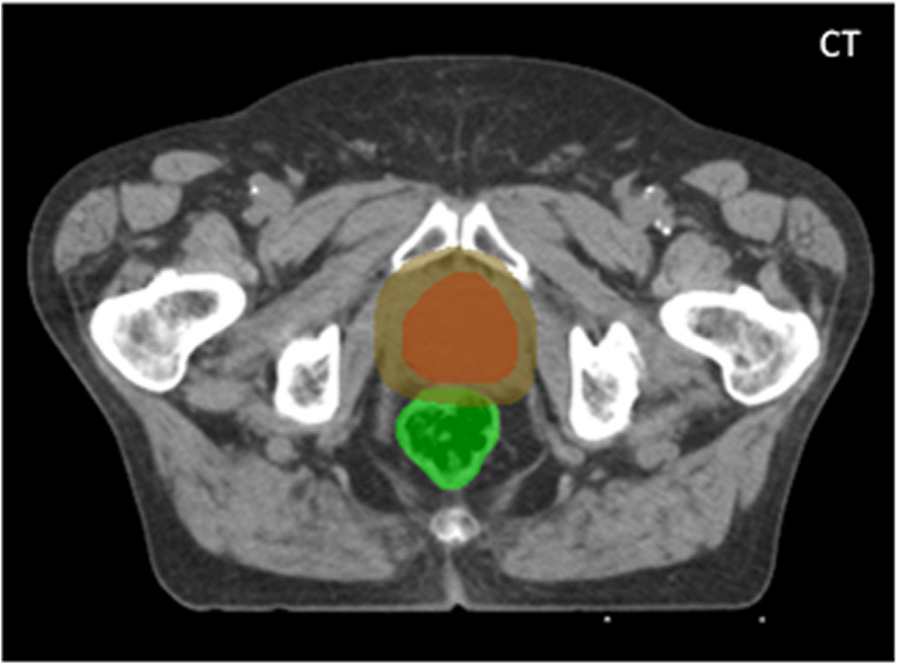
CT slice. Slice of a CT scan. The manual contours of (red) the prostate and (green) the rectum, as well as (brown) the ROI defined as the prostate gland extended with a margin of 8 mm are drawn. The rectal distension occurred between the CT and the CBCT scans was estimated by calculating the value of *F* as defined in Equation 2. *Ī*
